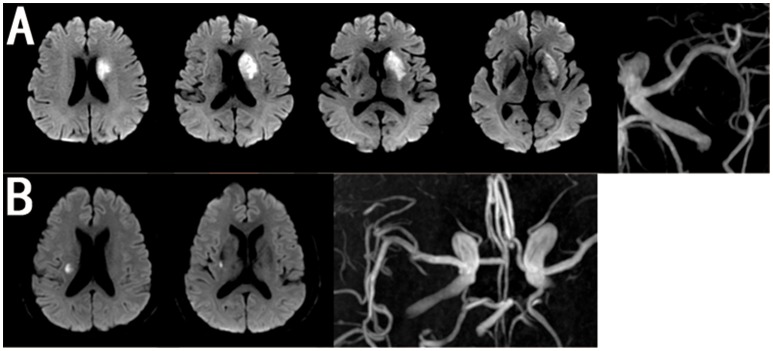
Representative MRI Images of BAD and LI in MCA. (A) BAD in anterior circulation with MRA showing none moderate or severe stenosis in ipsilateral MCA. (B) LI in anterior circulation with MRA showing none moderate or severe stenosis in ipsilateral MCA.
